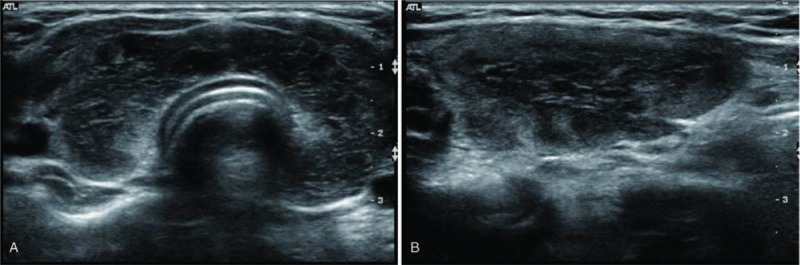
(A) Transverse and (B) longitudinal ultrasonographic images of Grade 4.
